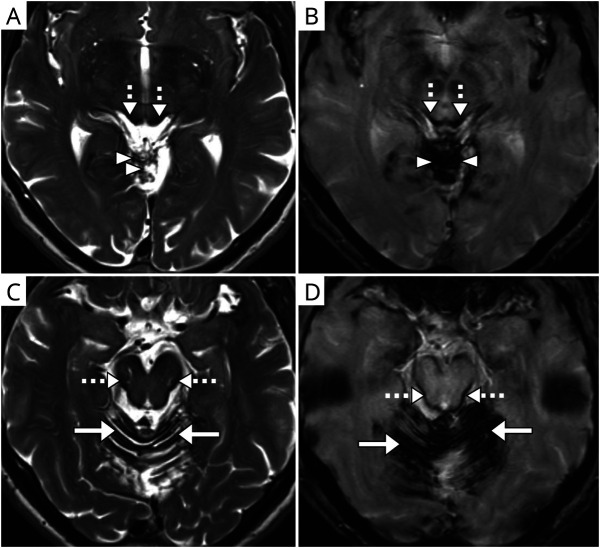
Axial Magnetic Resonance Images Showing Typical Appearances of Hemosiderin in iSS T2-weighted (A, C) and corresponding paramagnetic (B, D) sequences; images demonstrate the involvement of infratentorial regions: superior vermis (arrowheads, A, B), cerebellar folia (solid arrows, C, D), and midbrain (dashed arrows, A–D).
